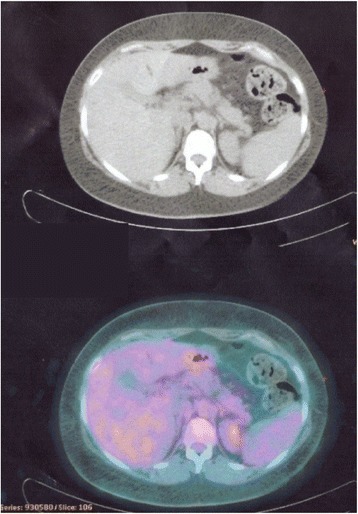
CT/PET scan showing CR in the liver lesions
